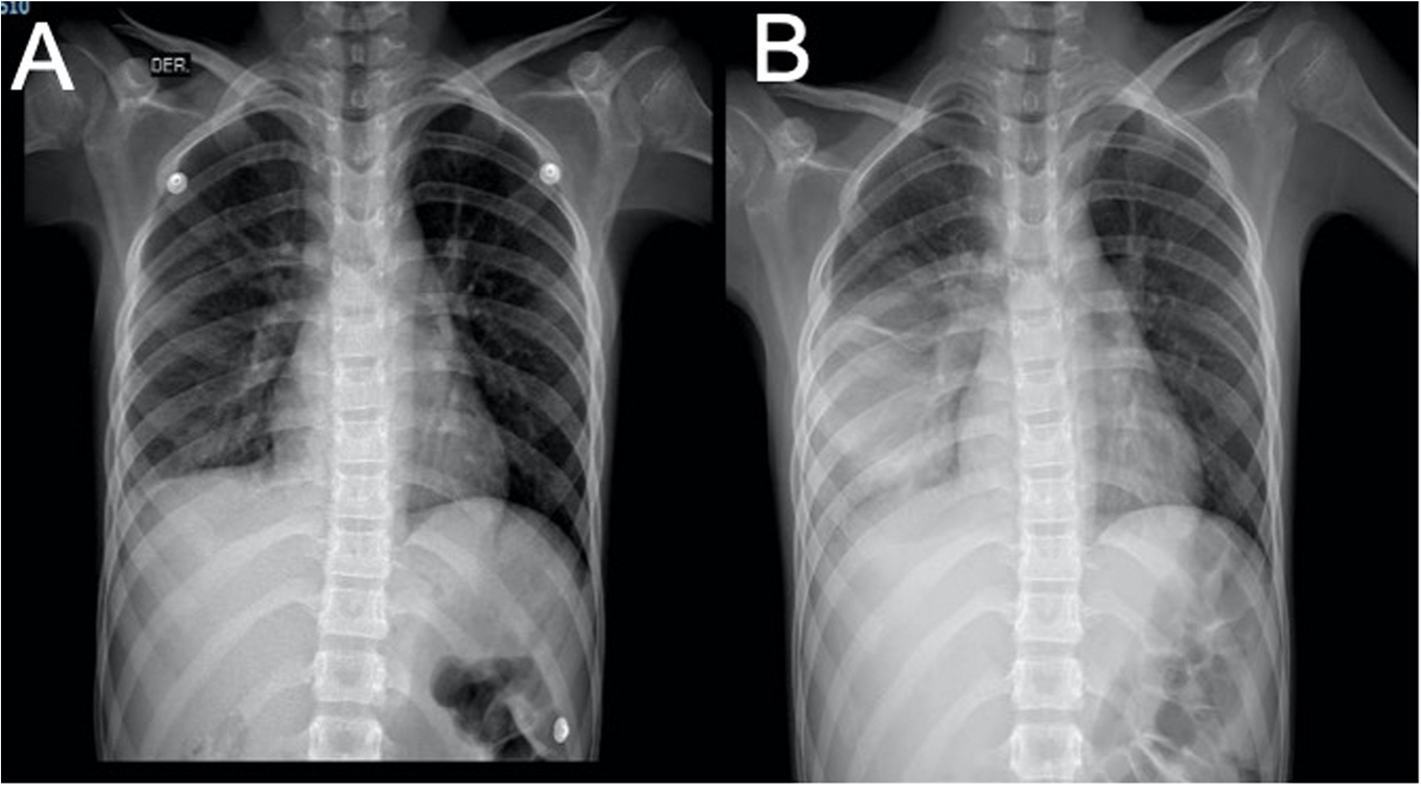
a) Radiography taken at admission with presence of right pleural effusion. b) Right basal consolidation and pleural effusion
